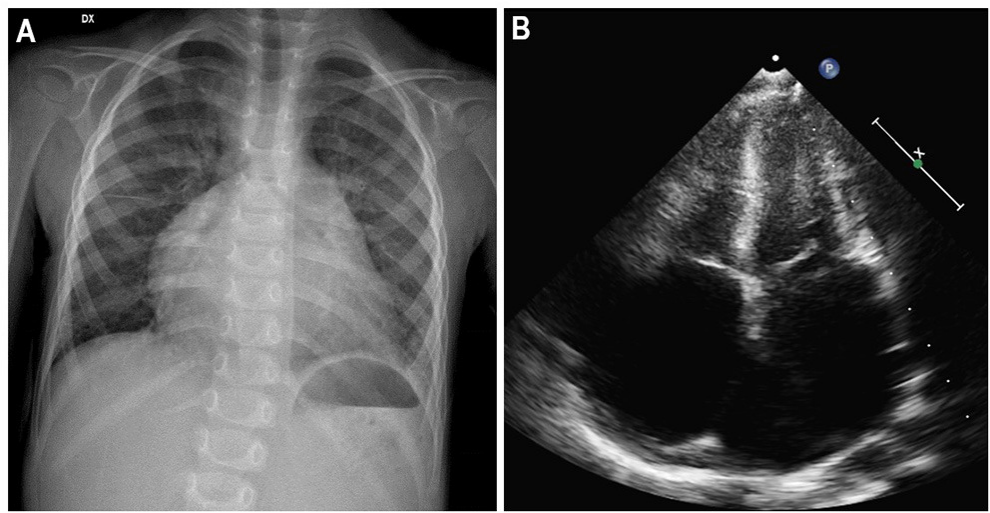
Three-year-old girl affected by restrictive cardiomyopathy. Antero-posterior chest radiograph (A) showing massive cardiomegaly due to severe biatrial enlargement, as confirmed by apical four-chamber echocardiography (B).
